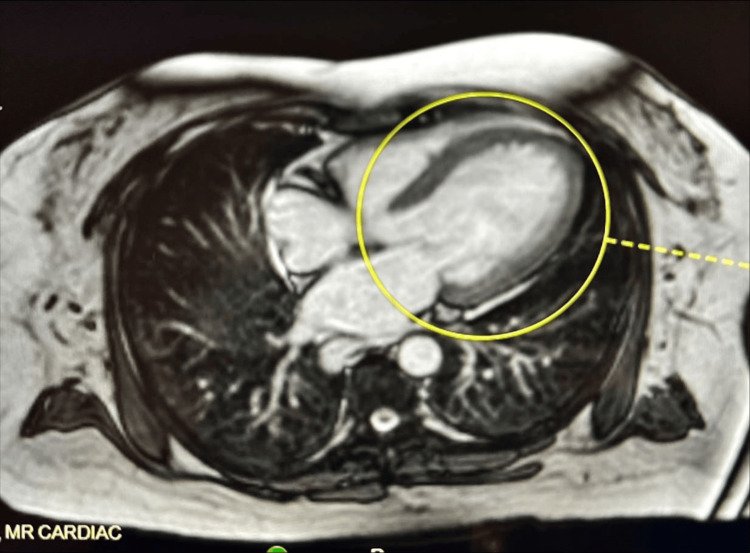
Cardiac MRI from day three of admission; axial true fast imaging with steady-state free precession of the whole chest (flip angle 80°). The left ventricle is encircled in yellow. Circle: Mixed concentric and eccentric left ventricular hypertrophy was appreciated
